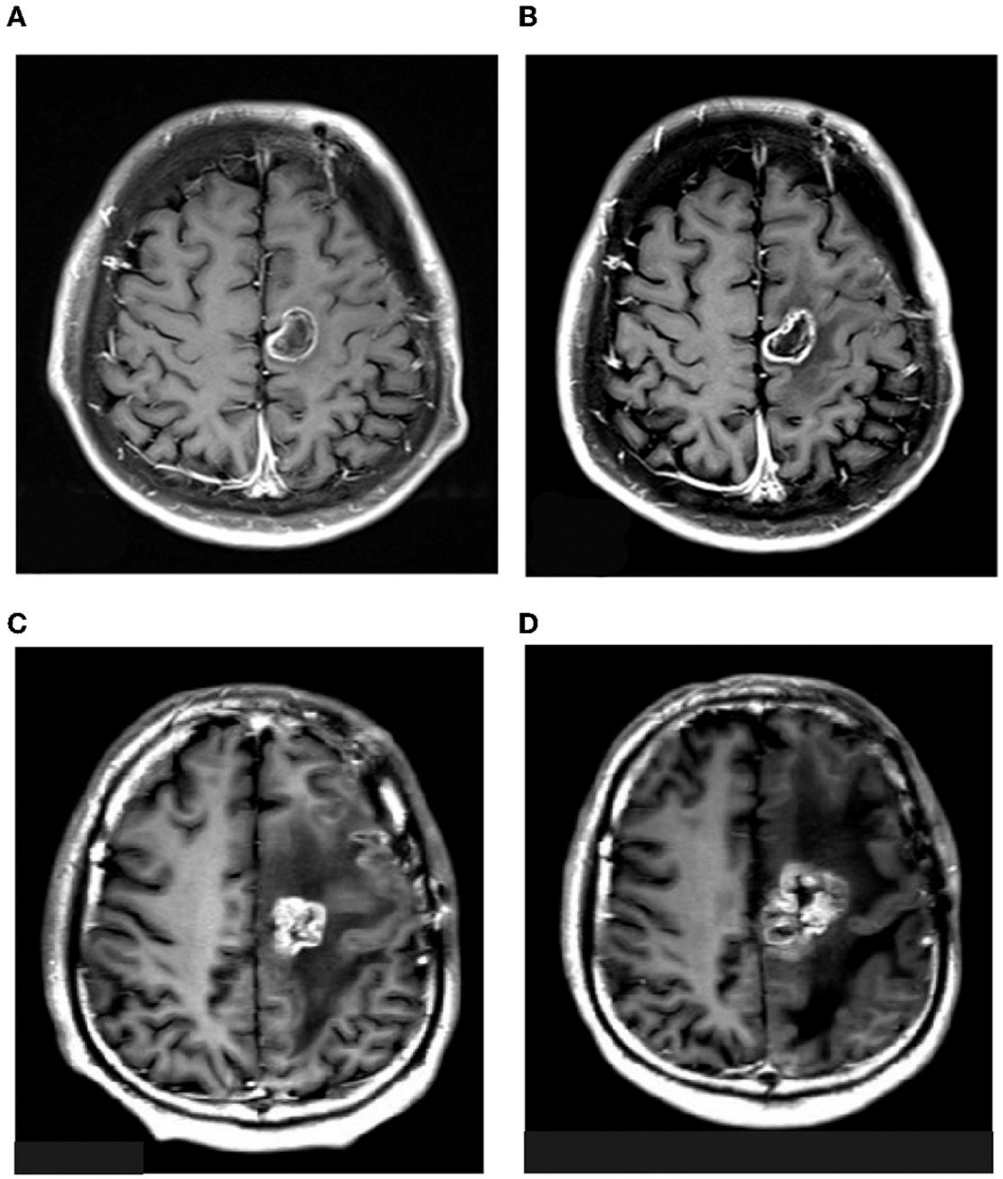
MRI images of the patient on 3rd March (A), 21st May (B), 4th August (C), and 15th November (D) 2021.
